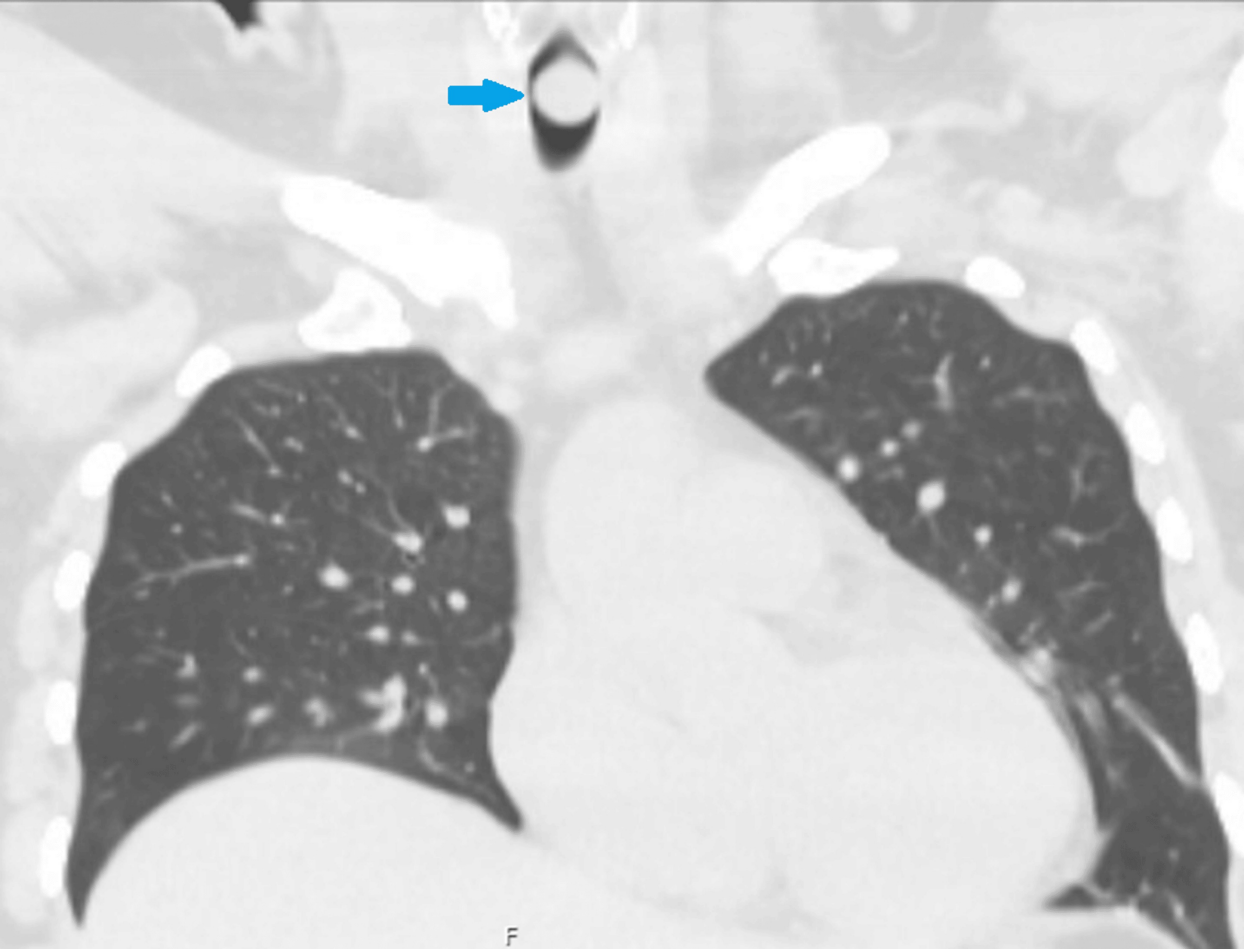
CT chest (lung window) of coronal cuts. Demonstrates severe tracheal narrowing secondary to a suspected tracheal mass (blue arrow).
